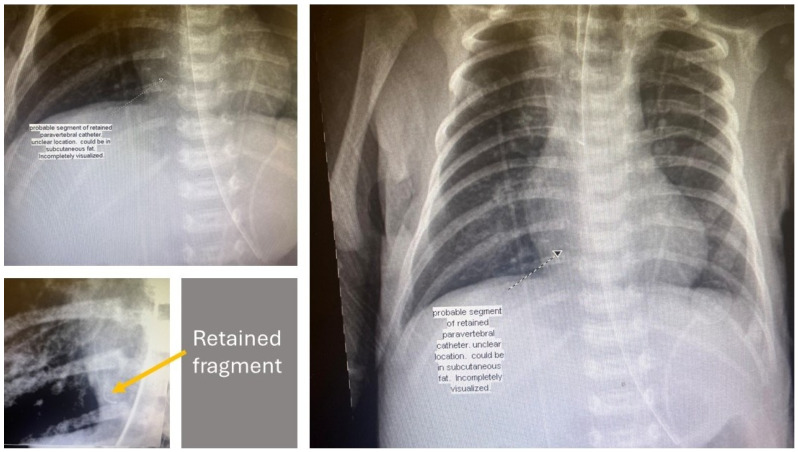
Chest X-ray revealing retained catheter fragment.
